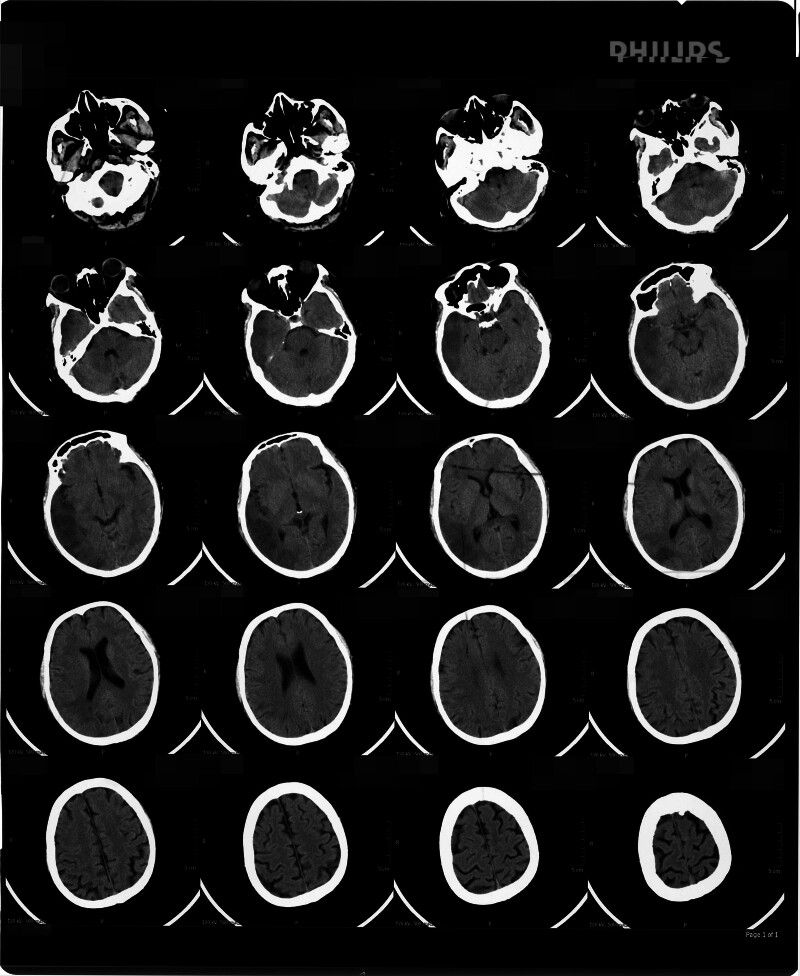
Cranial CT imaging demonstrated an extensive hypodense lesion involving the right temporo-parieto-occipital region on May 13, radiologically characteristic of acute cerebral infarction. CT = computed tomography.
